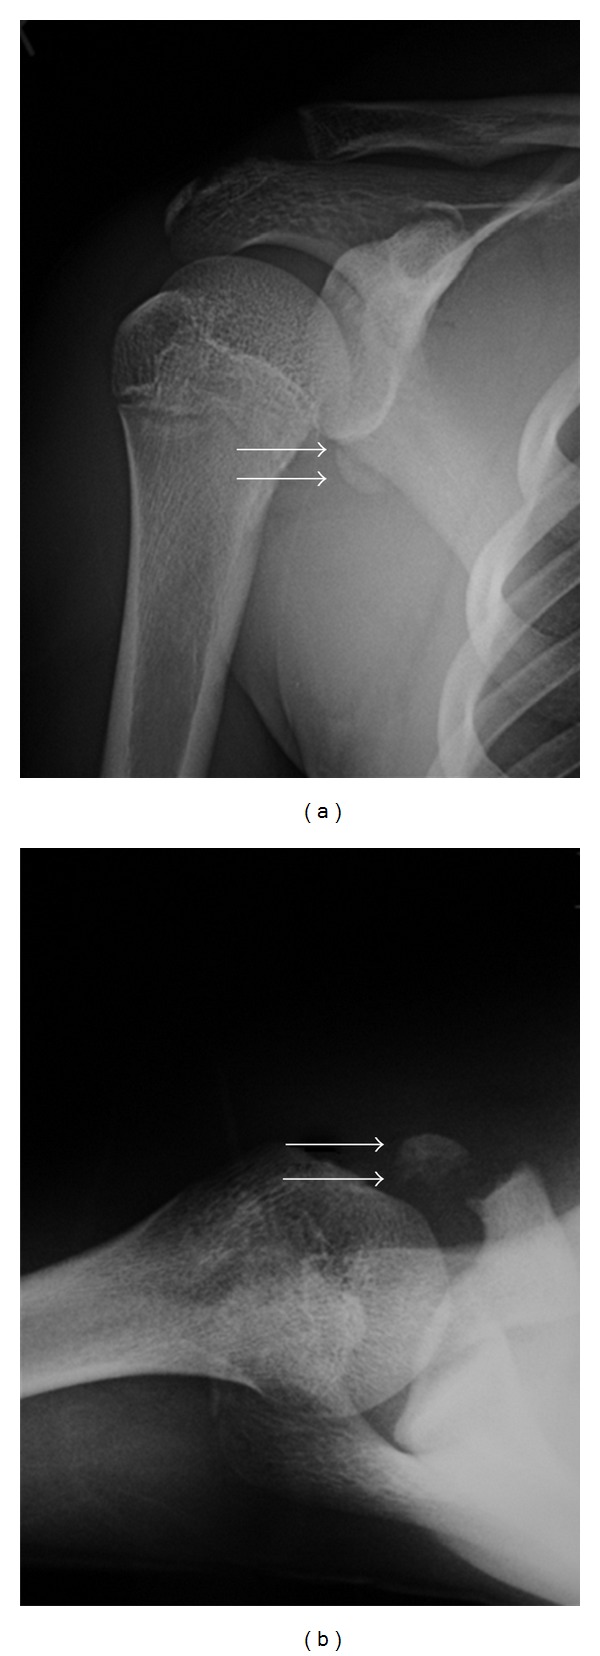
Plain radiographs showing epiphyseal fracture at the origin of the conjoined tendon on the coracoid process. White arrows indicate displaced fragment with coracobrachialis. (a) Anteroposterior view. (b) Axillary view.
